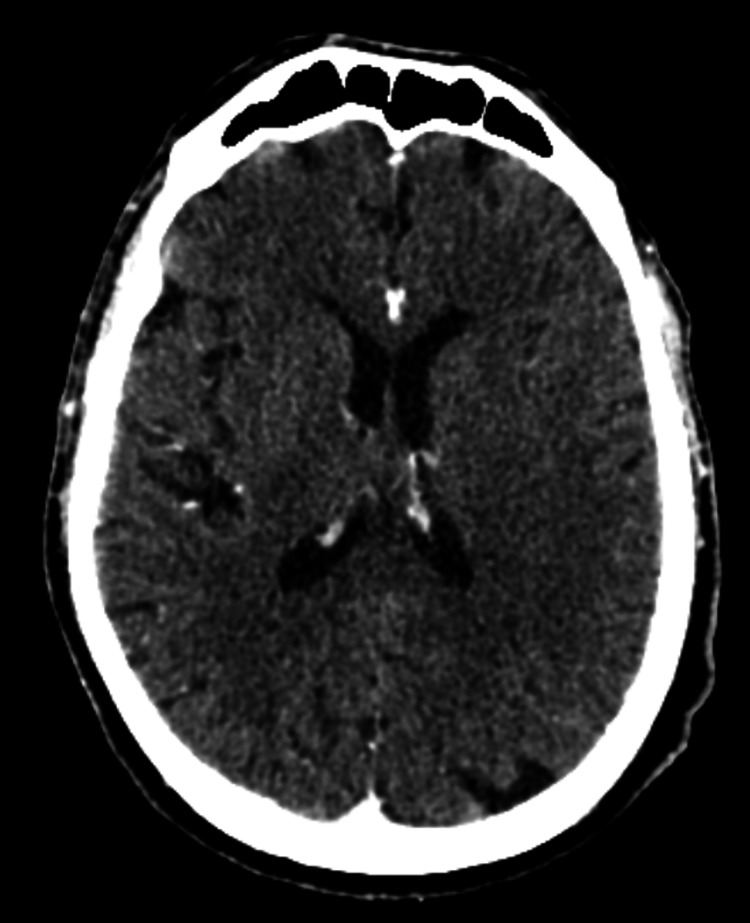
Brain CT scan showing no metastatic disease or acute findings (Case 2) CT: computed tomography
